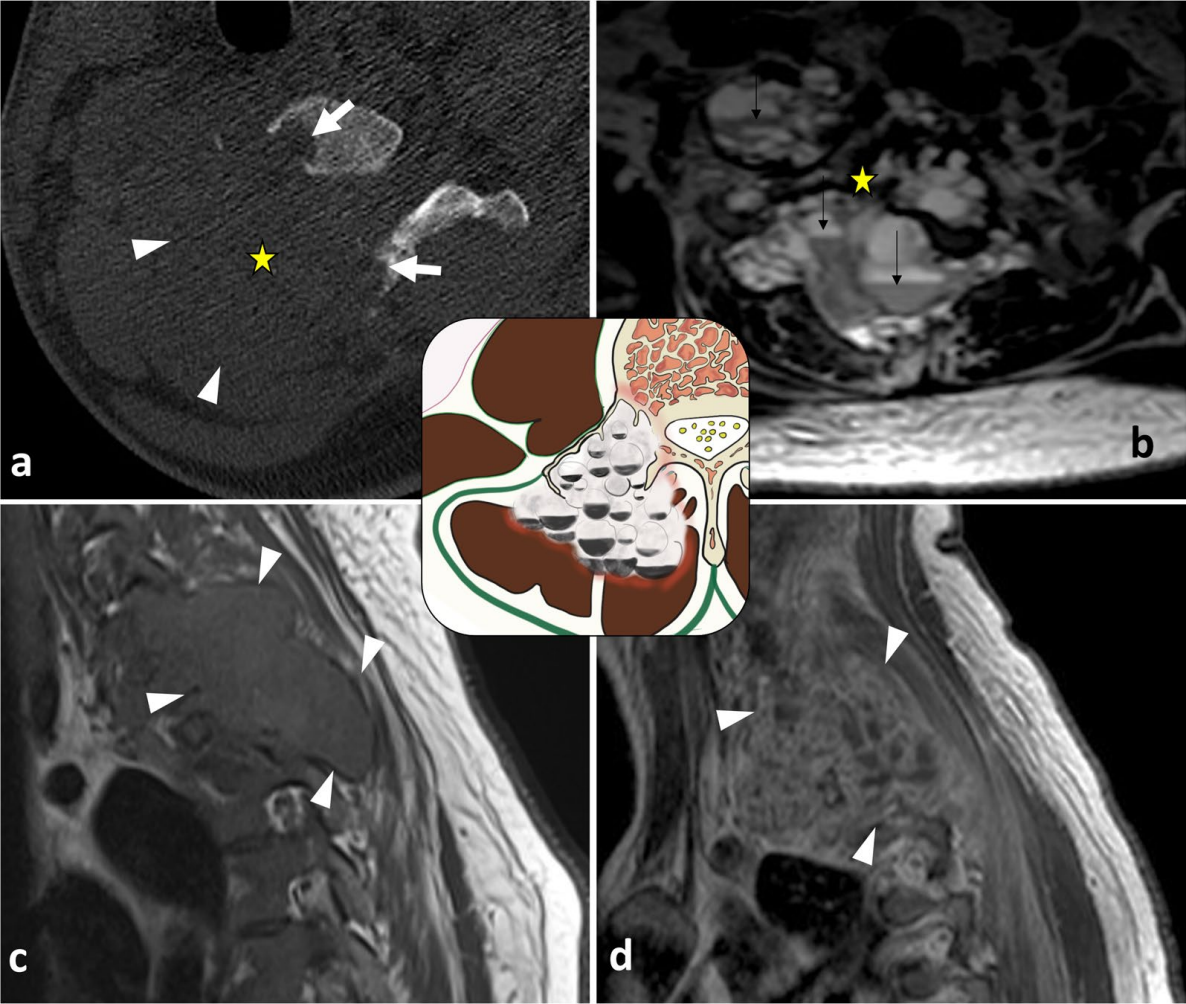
Aneurysmal bone cyst* in a 27-year-old female with cervical pain and weakness of the right upper limb. Axial CT scan reconstruction (a) shows an expansile, lytic lesion within the right neural arch and the vertebral body of C6 (white arrows). The mass invades epaxial and hypaxial region (arrowheads). Axial T2WI (b) demonstrates fluid–fluid levels (black arrows). Sagittal T1WI (c) and contrast-enhanced T1WI (d) show cystic areas. The lesion involves two adjacent vertebral levels. The yellow star shows the epicentre of the mass
